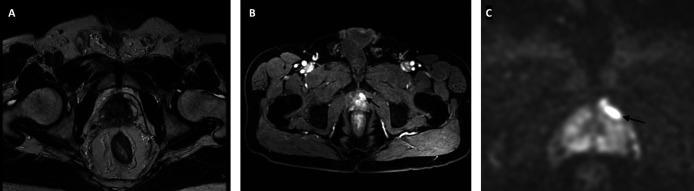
Multiparametric MRI of the prostate (A) T2-weighted axial image shows a homogenous hypointense lesion in the anterior zone of the prostate (B) Dynamic contrast-enhanced image shows contrast enhancement of the lesion; and (C) Diffusion-weighted image of the prostate.
